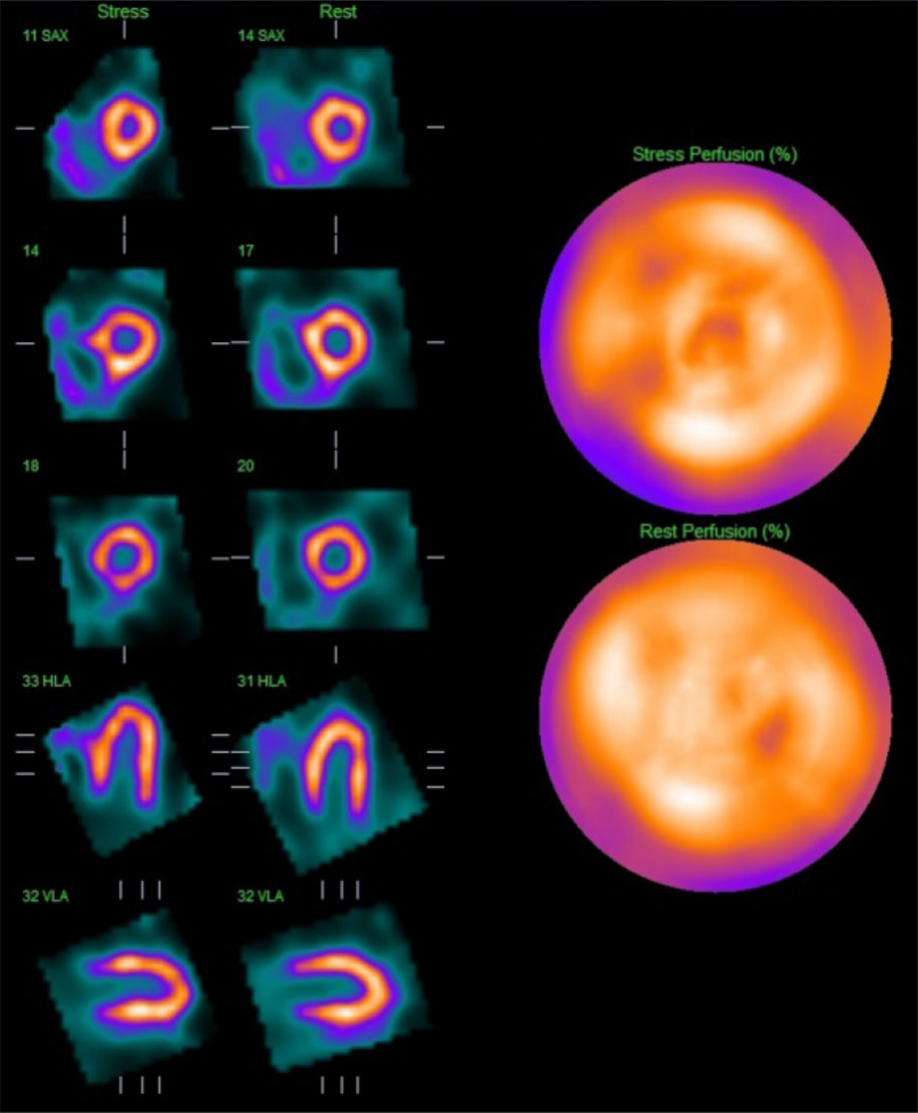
Case 2: Thallium-201 MPS of a 78-year-old man with a history of chronic respiratory disease complicated by recent type II respiratory failure showing no inducible perfusion abnormality. There is no significant inducible perfusion abnormality but the right ventricle is enlarged. *MPS* myocardial perfusion scintigraphy
